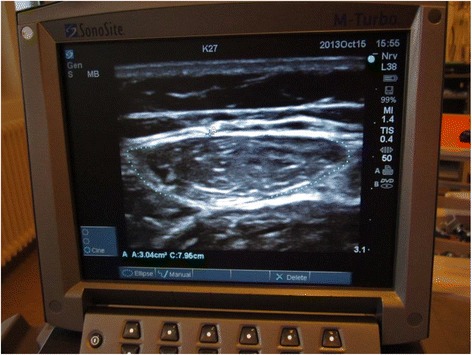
Ultrasonographic measurement of the rectus femoris muscle cross-sectional area. The rectus femoris muscle is part of the quadriceps muscle. Patients will be placed in supine position with their back raised to 45 degrees, with their legs in passive extension. The transducer will be placed over the rectus femoris muscle, perpendicular to the long axis of the right thigh, not depressing the dermal surface. Measurements will be made at 2/3 of the distance from the anterior superior iliac spine to the superior patellar border. This distance will be defined when the patient is placed as noted above, not with the patient standing up, since this changes the distance. For the scan, a linear transducer will be used, flat footprint, 5–8 MHz. The muscle is identified visually and an ultrasonographic picture is taken. Using the ultrasonographic software, the outer edge of the muscle is marked, and the cross-sectional area is calculated using planimetry
